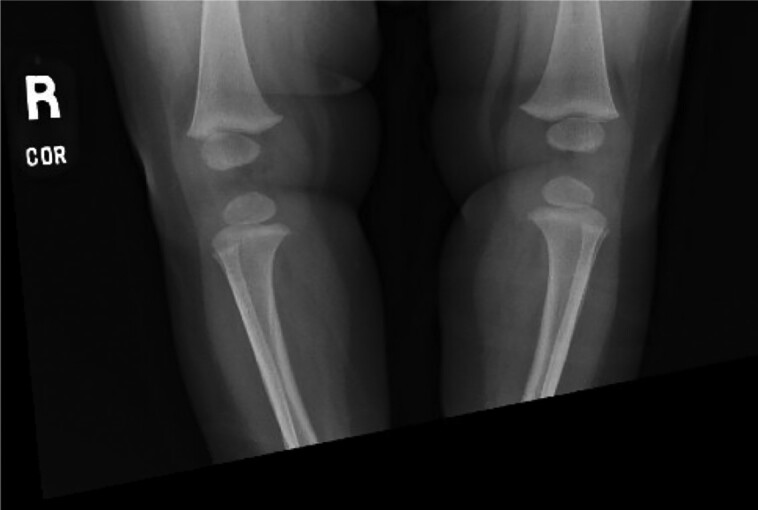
Radiograph of the bilateral lower extremities, age 8.5 months.
